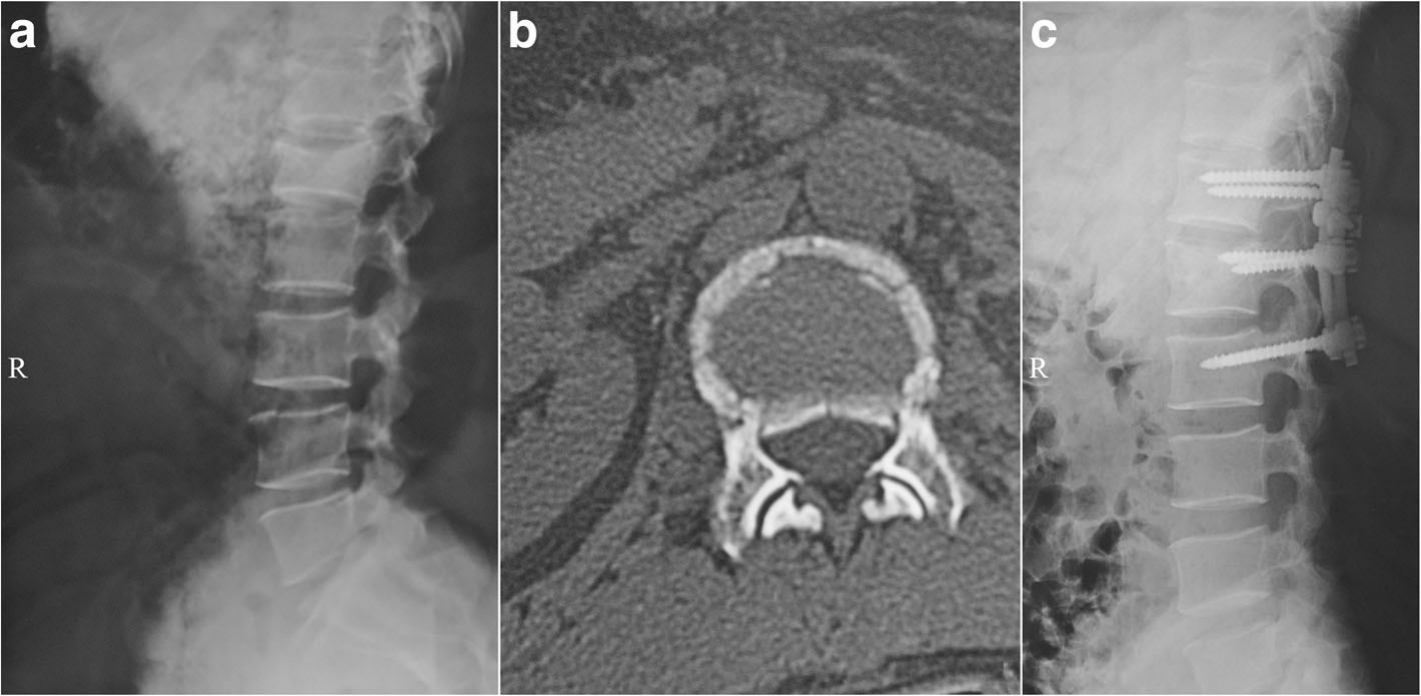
a Preoperative lateral radiograph of a 35-year-old female patient with a compression fracture at L1. b Preoperative CT of the patient. c Lateral postoperative radiograph of the patient
